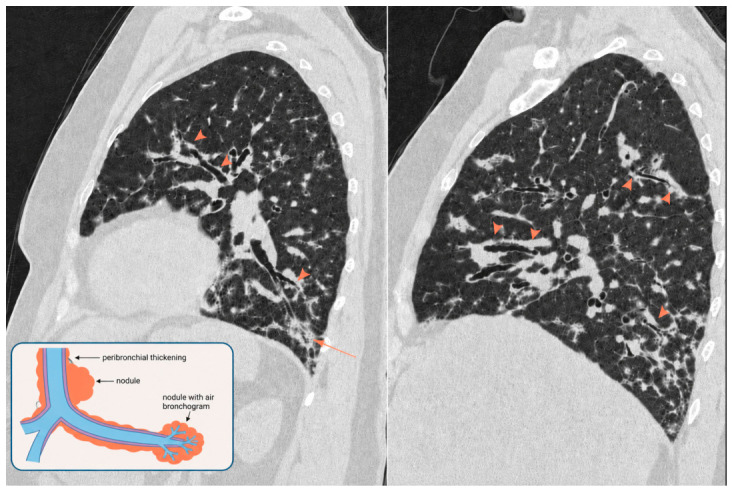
Sagittal non-contrast CT images show multifocal irregular (cylindric and nodular) peri-bronchial thickening, the Kebab sign (arrow head); the sign is better appreciated and should be sought on the sagittal plane; on the right, in the posterior costophrenic angle, a bronchocentric nodular consolidation is visible (arrow).
